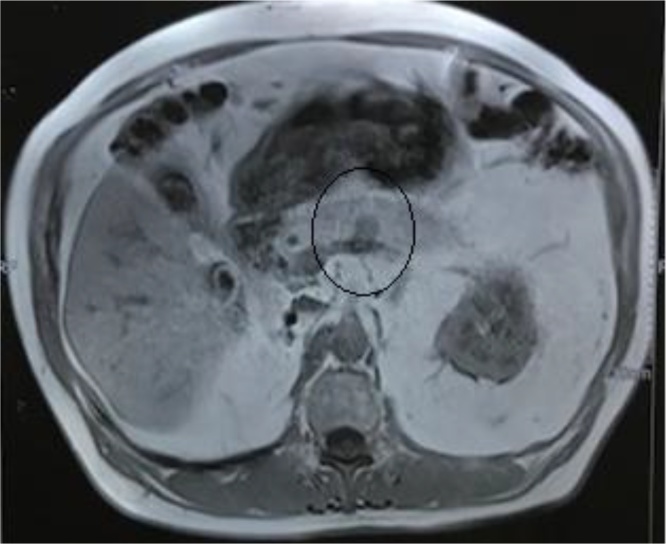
T1-weighted hypointense nodular formation in the body portion of the pancreas with 1.5 × 1.2 cm (circle).
